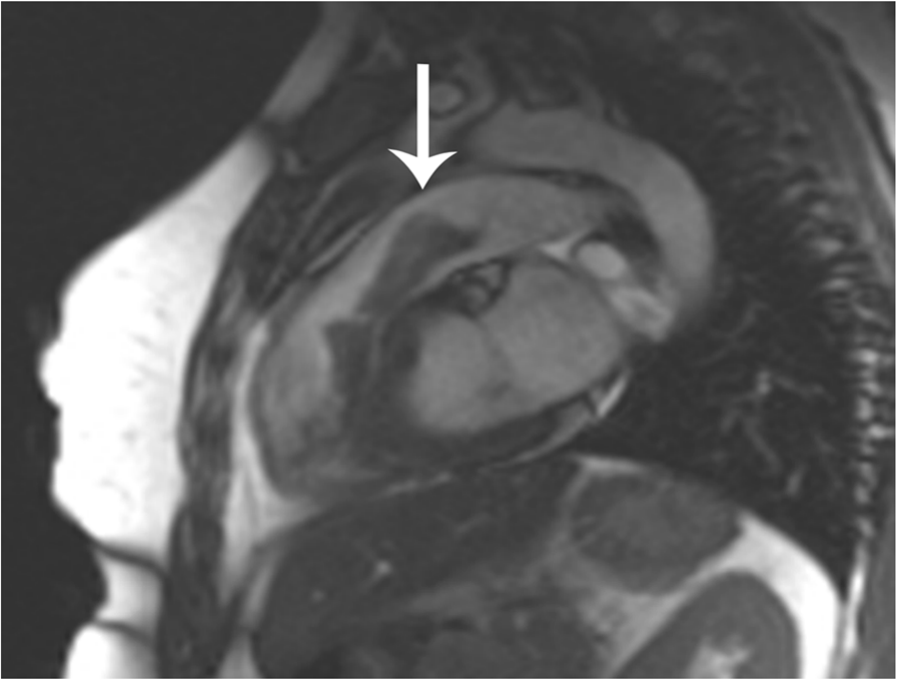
Cardiac magnetic resonance imaging in a sagittal plane demonstrating the piston-like shape of the thrombus, and how it protrudes through the pulmonary valve in systole
